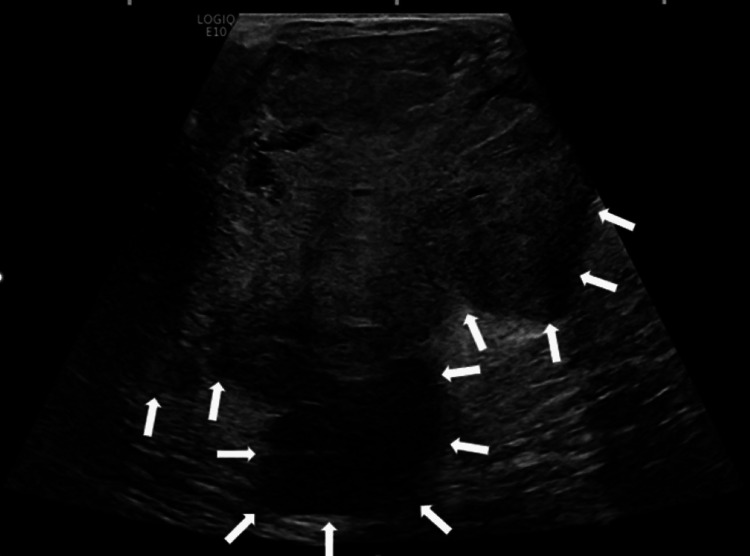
Right breast ultrasound of the patient. Right breast ultrasound showing large hypoechoic mass with small cystic components, internal vascularity, and posterior enhancement (area above the white arrows).
